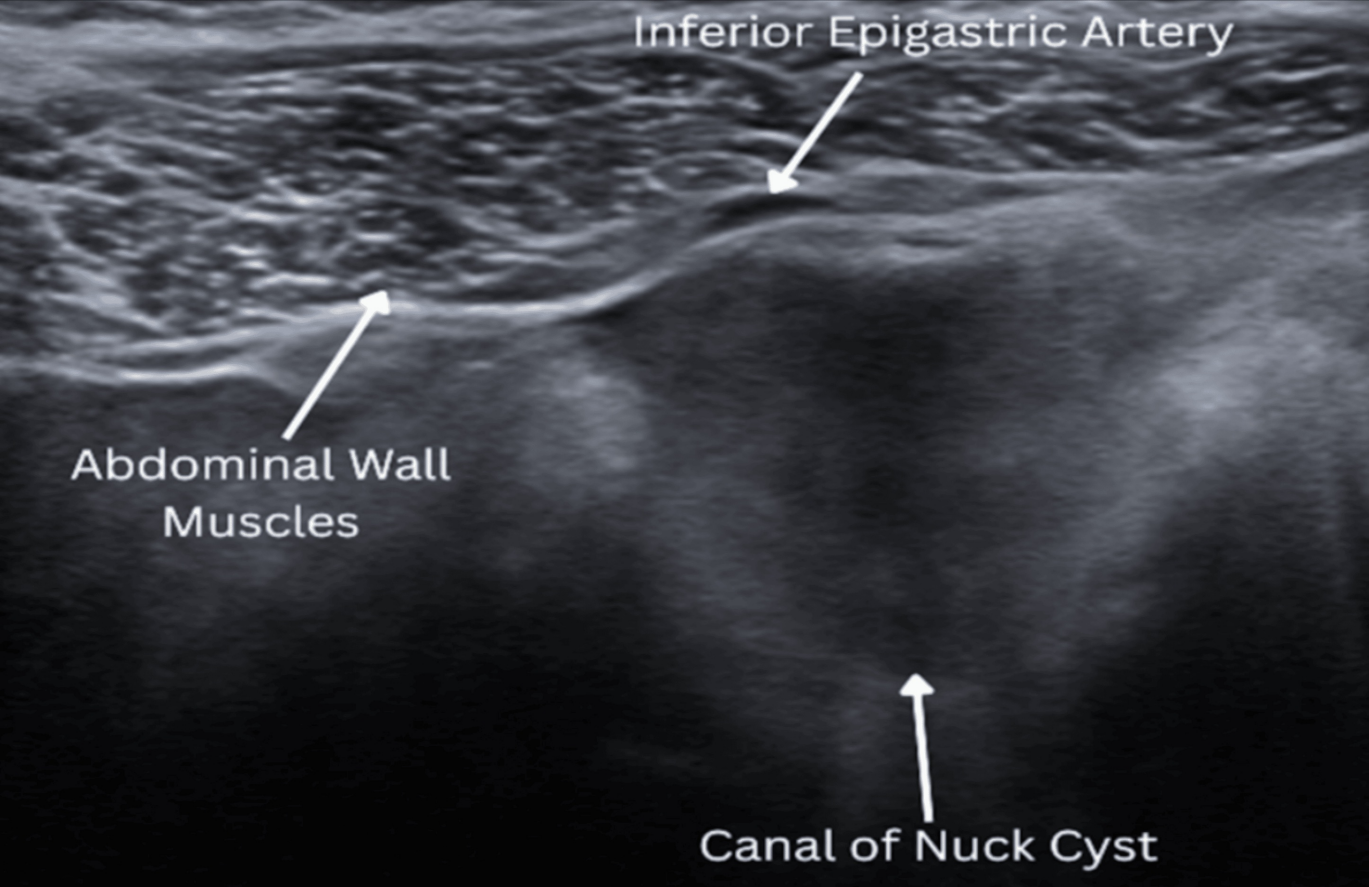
Ultrasound of a Canal of Nuck Cyst Ultrasound shows a 4.2 × 4.4 cm ill-circumscribed, fluid-filled structure in the right inguinal canal, representing a canal of Nuck cyst
